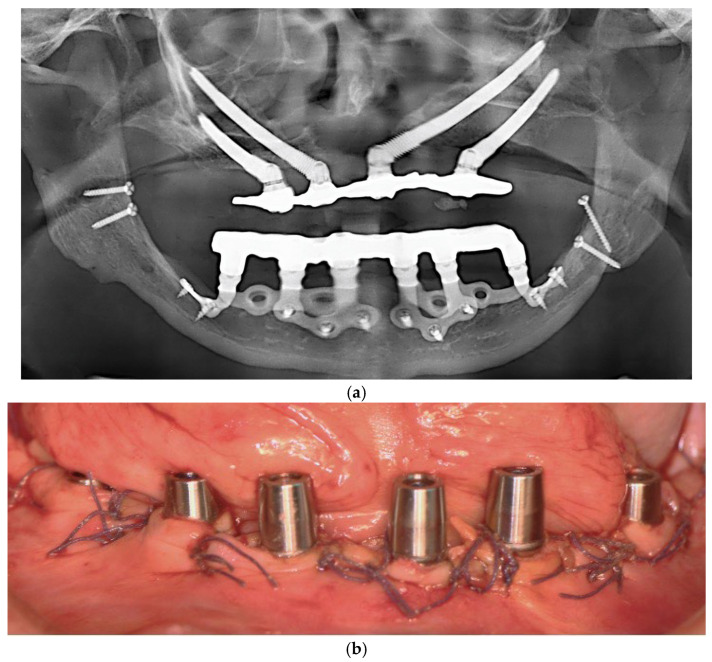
(a) A panoramic radiograph of the patient after subperiosteal implants placement. (b) Intraoral picture of the mandible following subperiosteal implant placement.
